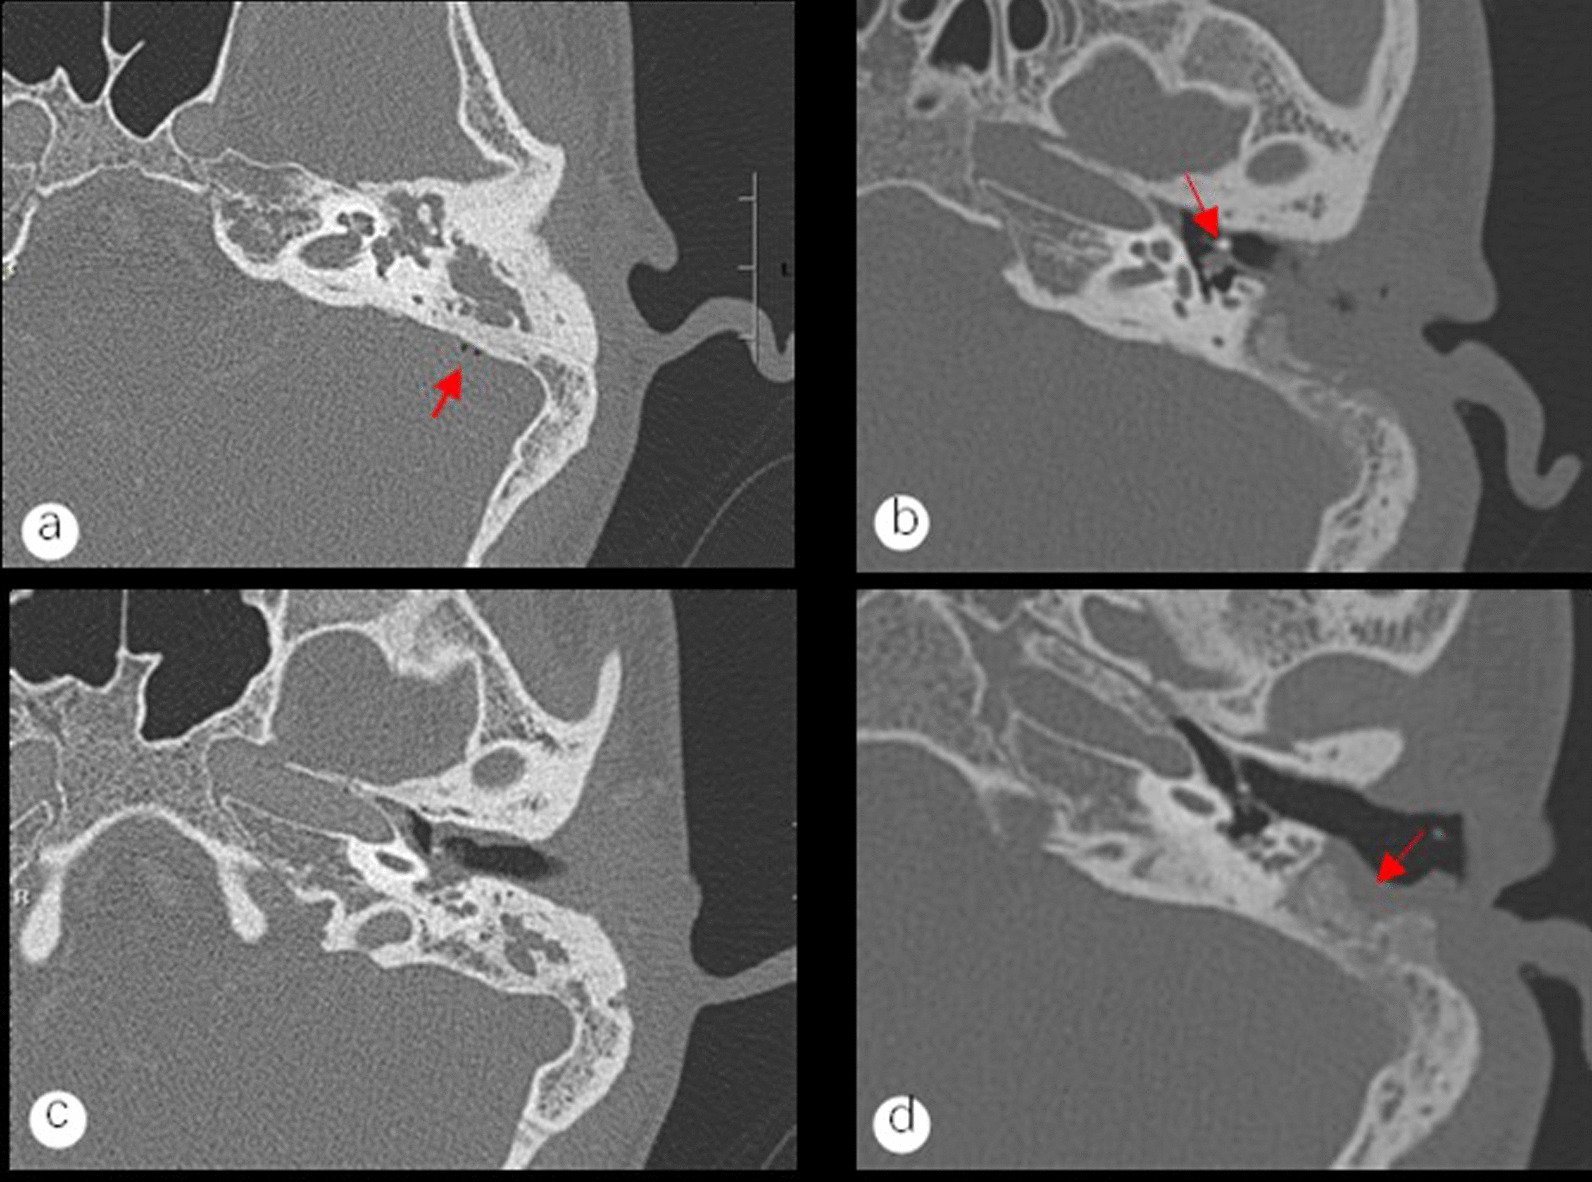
a The patient's preoperative axial temporal bone CT shows bone destruction of the long pedicle of the anvil, with the air shadow around the sigmoid sinus shown by the arrow. b The patient's temporal bone CT was repeated 3 months after tympanoplasty. The reconstructed cartilage piece of the auricular cavity shown by the arrow established contact with the vestibular window and isolated in the middle tympanic cavity. c The patient's preoperative axial temporal bone CT with soft tissue filling of the eustachian tube bullae. d The middle ear cavity was restored to contain air 3 months after surgery, and the reconstructed external auditory canal was in good shape. The arrow shows the grafted fascia and the filled bone powder, and the fascia has survived
